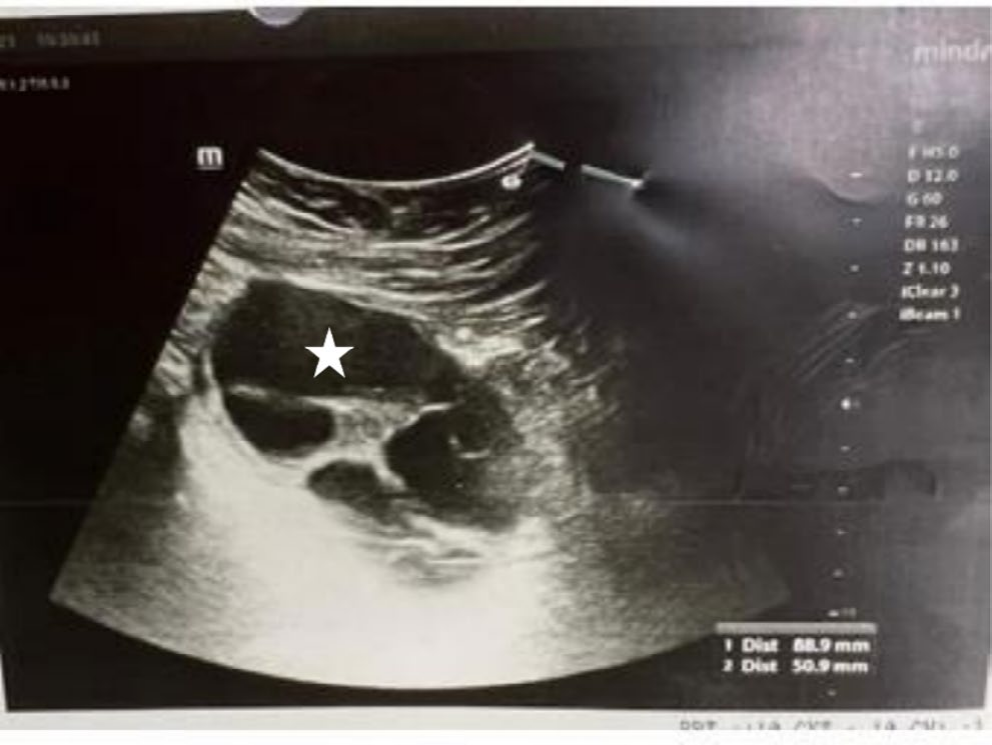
Ultrasound imaging demonstrating a multilocular cystic lesion in the left ovary, characterized by internal echogenic septa, prompting further evaluation to assess potential underlying conditions.
